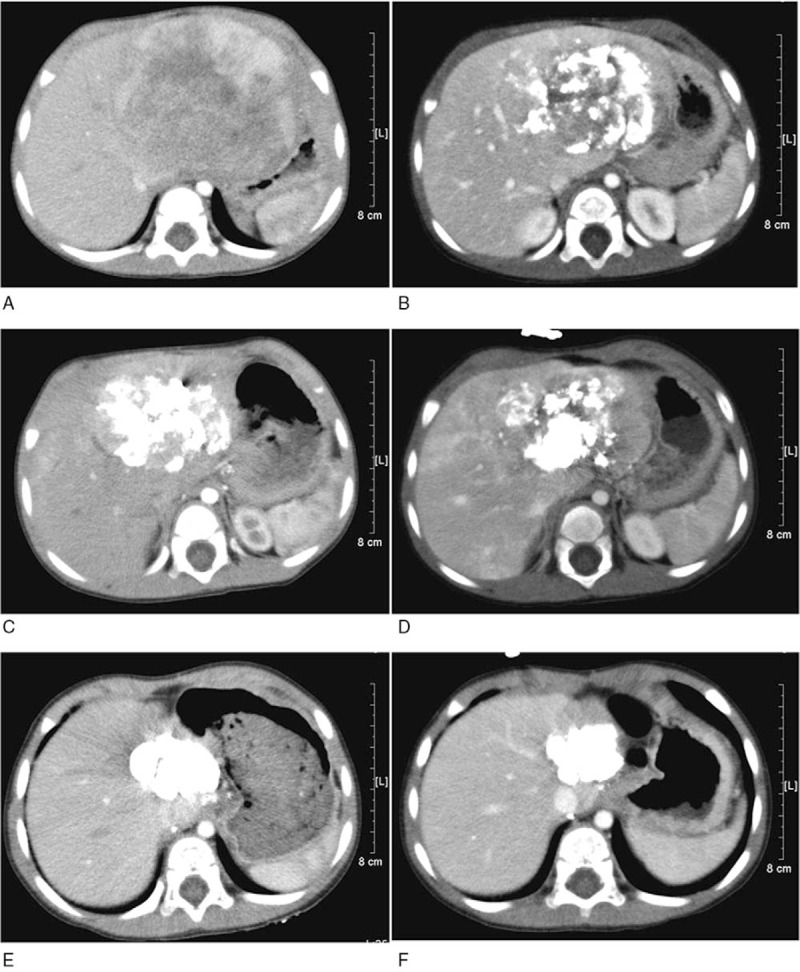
A 1-year-old boy with PRETEXT IV hepatoblastoma who had experienced 2 times of TACE, 1 time of MWA, and 4 cycles of chemotherapy. (A) Contrast-enhanced computed tomography (CT) scan showing a huge lesion in the liver. (B) Contrast-enhanced CT scan revealing that Lipiodol deposited within a part of the tumor after 1 time of TACE was performed. (C) Lipiodol deposited within most parts of the lesion in CT scan after 2 times of TACE. (D) Active lesion necrosis was observed in contrast-enhanced CT scan after MWA. (E) Contrast-enhanced CT scan at 6 months after MWA. The necrotic part of the lesion was absorbed while the other part of the lesion was deposited with high-density Lipiodol. (F) Contrast-enhanced CT at 15 months after MWA revealing that the tumor was absorbed gradually with no active lesion. CT = computed tomography, TACE = transcatheter arterial chemoembolization, MWA = microwave ablation.
